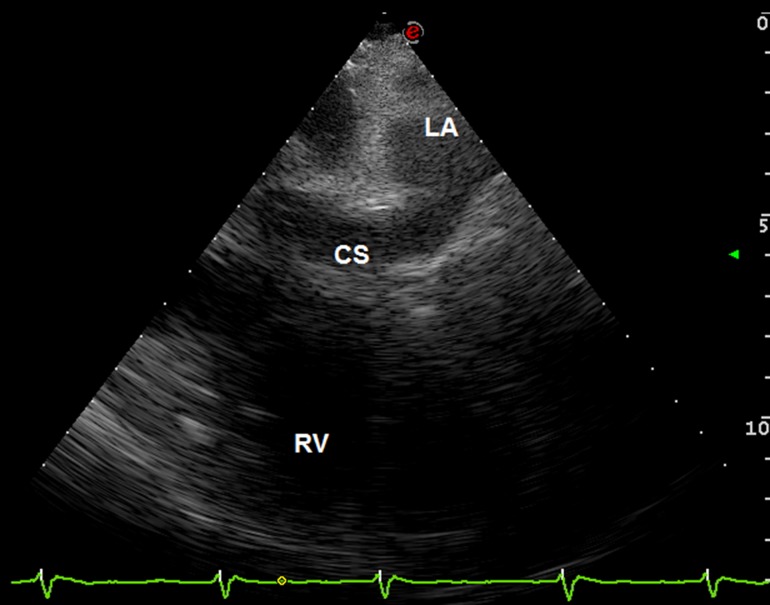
Transesophageal echocardiogram at 0 degree demonstrating the connection between the mid portion of coronary sinus and left atrium Abbreviations: CS: Coronary Sinus; LA: Left Atrium; RV: Right Ventricle
